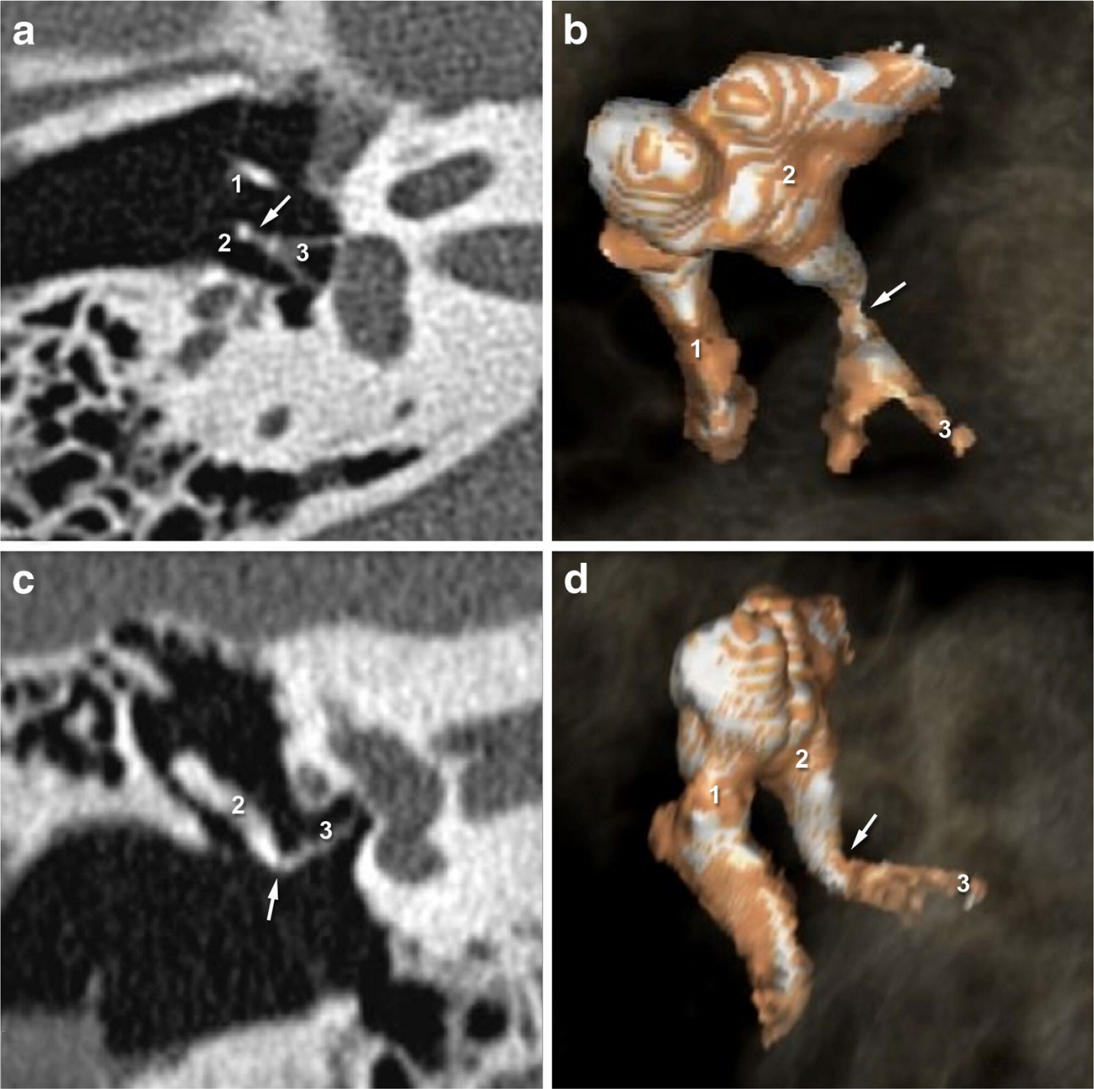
2-D CT images in the axial and oblique planes showing a normal incudo-stapedial joint. The malleus is annotated (1) The axial and oblique planes (a and c) show the ossicular « V », comprising the long process of the incus (2) and the stapes (3). 3-D-CT acquisition (b and d) reveal continuity between the stapes head and the long process of the incus in the healthy middle ear
